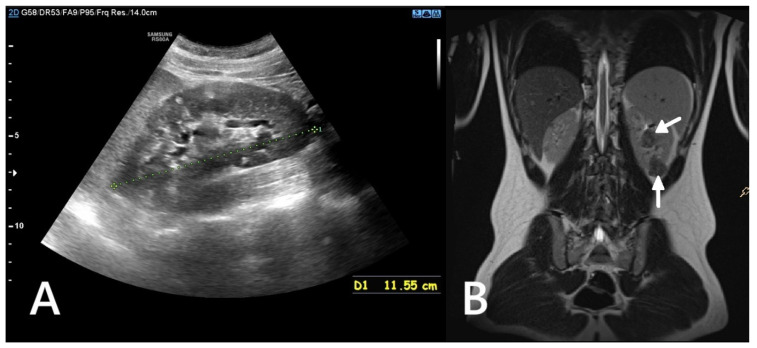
Ultrasound (A) and MRI (B) images of a 16-year-old boy. The ultrasound reveals numerous hyperechoic lesions, consistent with AMLs, along with several cysts (A). MRI shows large focal lesions (arrows), most likely representing atypical AMLs (B).
